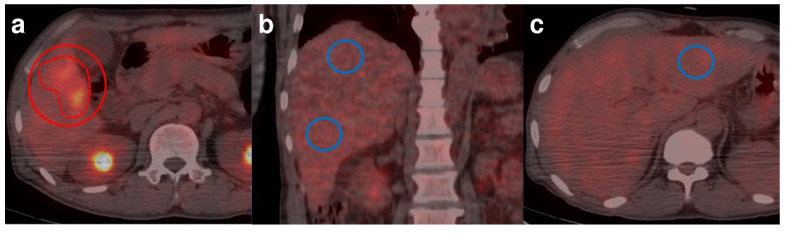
Measurement methods on ^18^F-fluorodeoxyglucose positron emission tomography/computed tomography images. (a) A spheric volume of interest (VOI) was carefully drawn to include the entire tumor (solid line), and the tumor contour (dashed line) was automatically drawn using a specific threshold of 41% of the maximum value. Then the maximum standardized uptake value (SUV) normalized by total body weight and the maximum SUV normalized by lean body mass was obtained. (b,c). For the background-liver regions, three spherical VOIs (14 cm^3^) of 30-mm diameter (blue circle) were drawn at 3 sites of the liver, 2 above and below the right portal vein, and 1 at the middle level of the left lobe, in a region where the tumor was not detected on other images.
